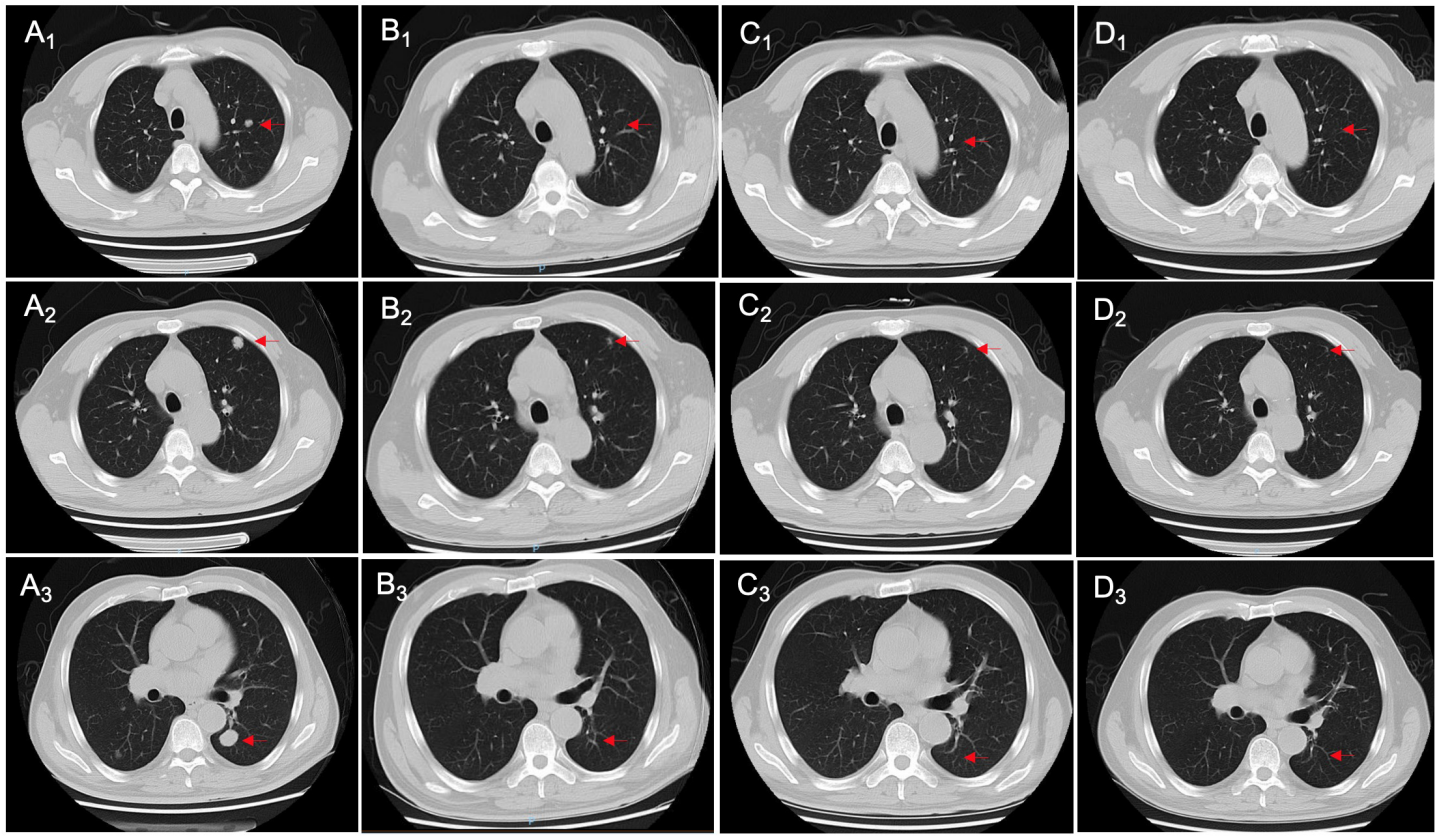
Changes in chest CT imaging of patient 2 during treatment, including initial diagnosis (A_1_-A_3_), post-neoadjuvant chemotherapy (B_1_-B_3_), 3 months after radical cystectomy (C_1_-C_3_), and 6 months after radical cystectomy (D_1_-D_3_). The red arrows point to the nodules.
